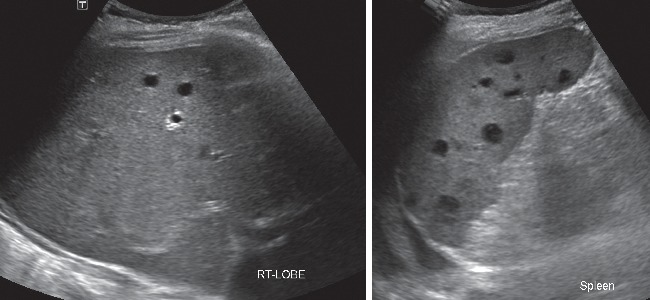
Ultrasound showed multiple hypoechoic nodules in the liver and spleen, compatible with abscesses.
